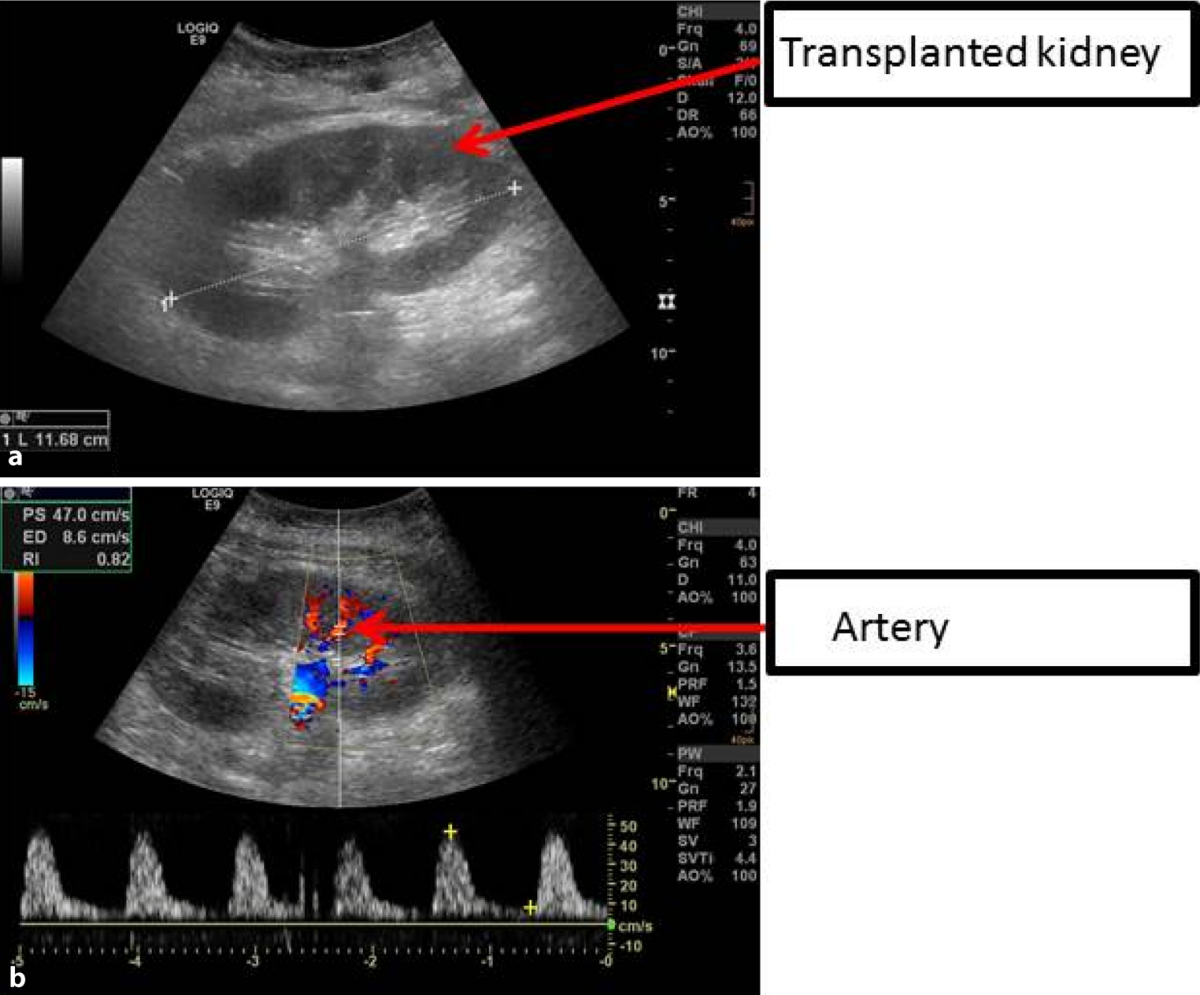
a Representative ultrasound image of a transplanted kidney. b Normal resistance index is measured in an interlobar artery
